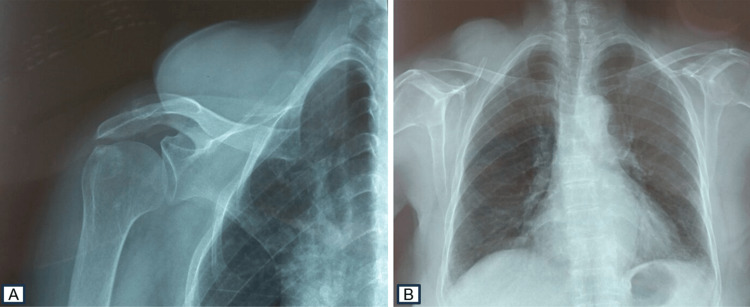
Increased soft tissue contours on the shoulder X-ray with a normal bone skeleton (A, B).
